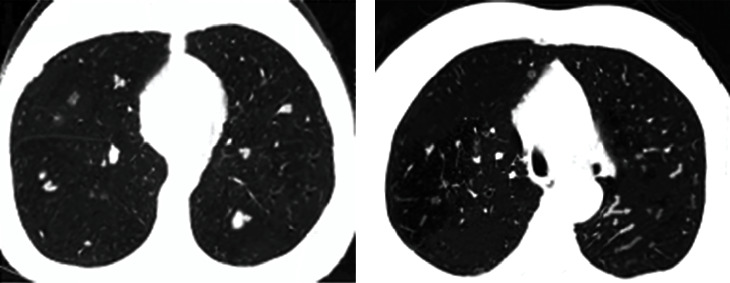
CT images of different types of patients. (a) Man, 57 years old, with an in-situ adenocarcinoma of the upper lobe of the right lung; (b) Man, 57 years old, with a microinvasive adenocarcinoma of the middle lobe of the right lung.
